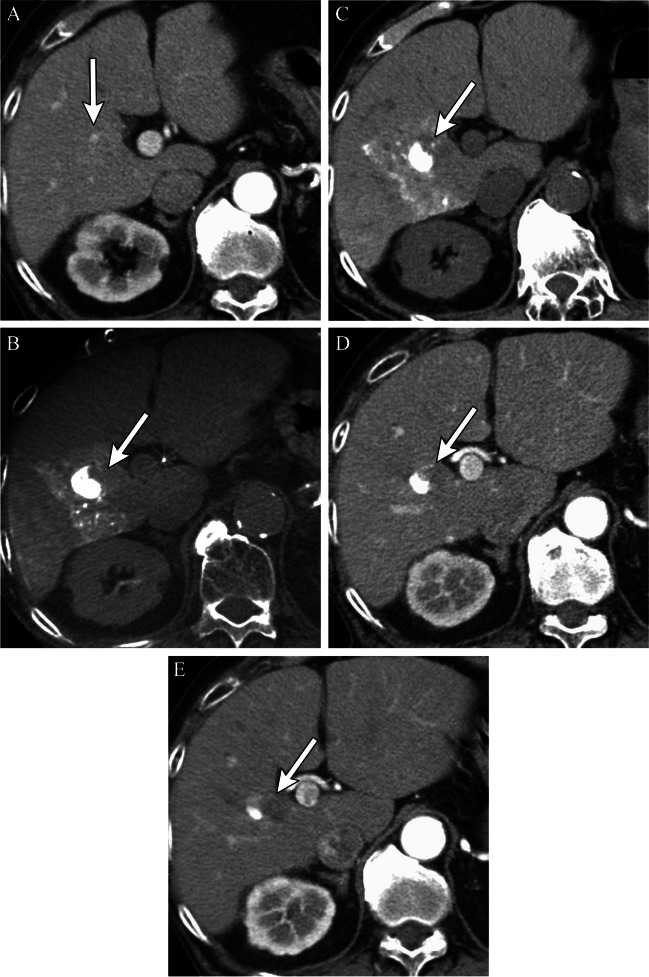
Defect of Lipiodol accumulation in the tumor on CBCT after TACE. 56-year-old with cirrhosis. Arterial-phase CT shows a small tumor (arrow) (A). TACE was performed, but CBCT was performed immediately after TACE demonstrated a defect of Lipiodol accumulation in the tumor. Another feeder was searched, but it could not be identified (B). Unenhanced CT performed 1 week after TACE also showed a defect of Lipiodol accumulation in the tumor (C). Arterial-phase CT performed 2 months after TACE revealed nodular APHE along the margin, compatible with viable tumor, LR-TR Viable (D). Five months post cTACE with no interval treatment, there is continued local progression with increasing size of the nodular perilesional tumor, LR-TR viable (E)
